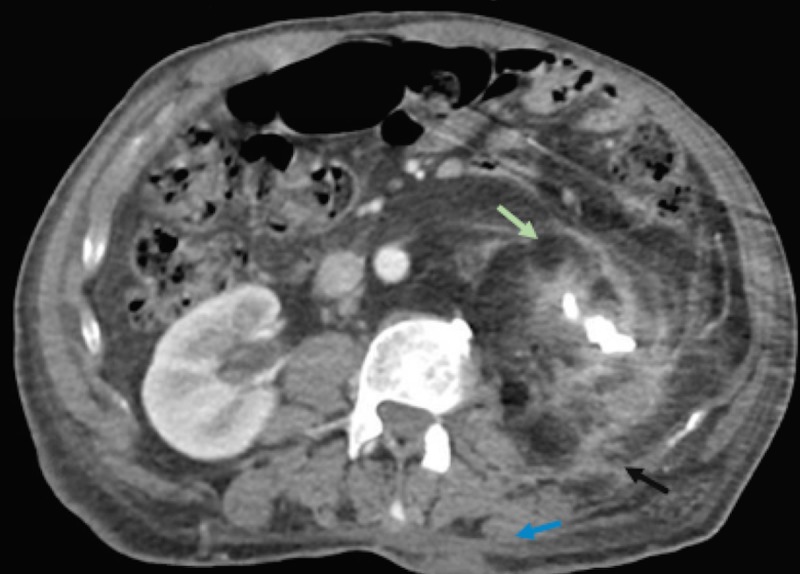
CT scan of the abdomen and pelvis displaying an atrophic left kidney (green arrow), fistula to the adjacent tissue (black arrow), as well as a fistula to the skin (blue arrow) CT: computed tomography
